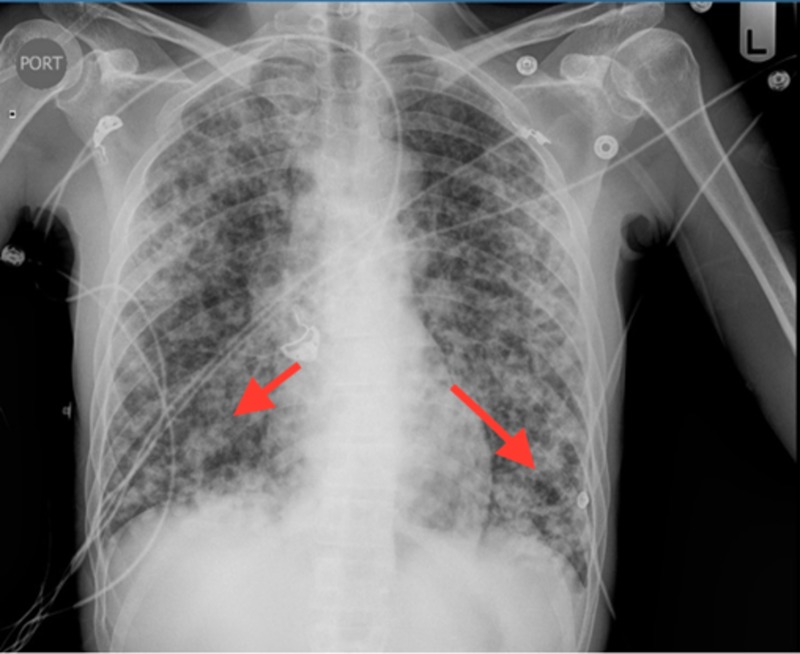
Chest x-ray revealing bilateral coarse reticulonodular opacities and cysts
